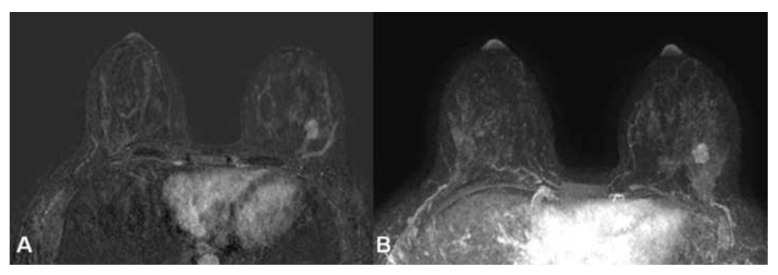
Subtracted THRIVE (A) and 3D MIP (B) contrast-enhanced MRI showing a NST breast cancer on the left side in a 44-year-old HL survivor with Type 3 BPE evaluated in the contralateral breast.
